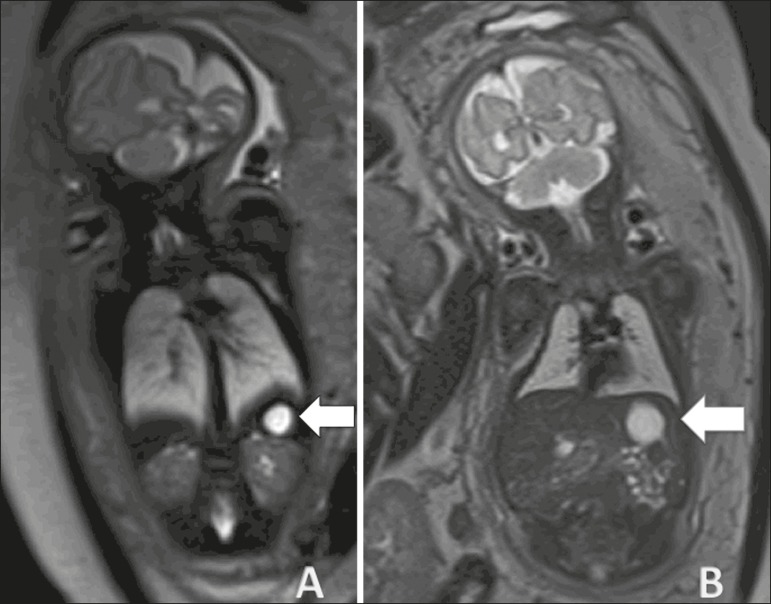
Splenic cyst. A: Coronal T2-weighted sequence of a fetus at
